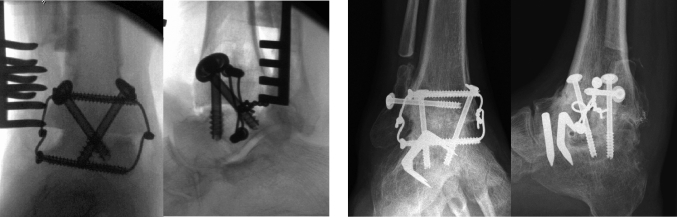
A. Fluoroscopic perioperative images of the right ankle in a patient after revision ankle arthrodesis by use of a 4 cannulated screw fixation technique. The ankle arthrodesis healed after 10 weeks. B. Radiographs 9 months after surgery in a patient with congenital clubfoot deformity previously treated by attempted ankle arthrodesis and subtalar arthrodesis with union.
